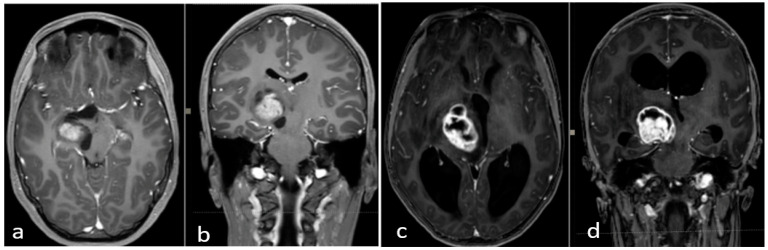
Preoperative brain MRI of the thalamopeduncular tumor of Case A (a,b) and of Case B (c,d). (a,b) Brain MRI, T1-weighted with gadolinium, showing solid-cystic right thalamopeduncular tumors in the axial plane (on the left) and coronal plane (on the right) in Case A. (c,d) Brain MRI, T1-weighted with gadolinium, showing a right thalamopeduncular lesion with disomogenous contrast enhancement in the axial (on the left) and coronal (on the right) plane in Case B.
